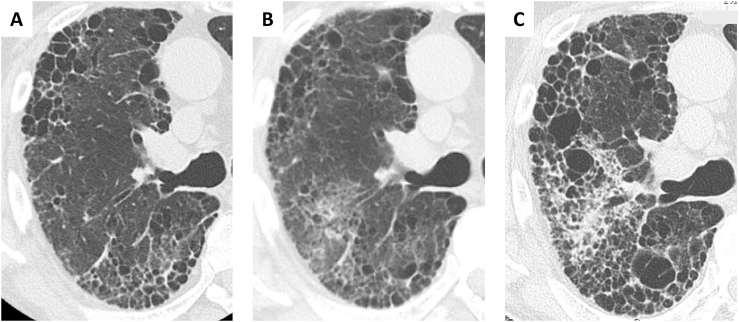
Serial changes of high-resolution CT findings before the diagnosis of acute exacerbation (AE) of idiopathic pulmonary fibrosis (IPF). (A) At the diagnosis of IPF (B) At the diagnosis of coronavirus disease 2019 (C) At the diagnosis of AE of IPF.
